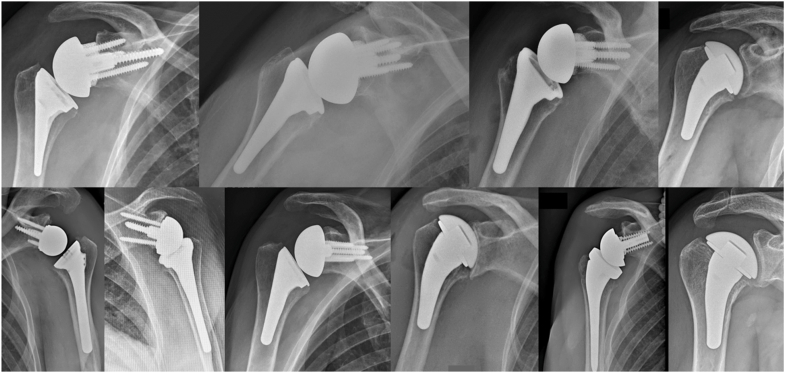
Post-operative radiographs of the first 10 cases treated with this approach.
